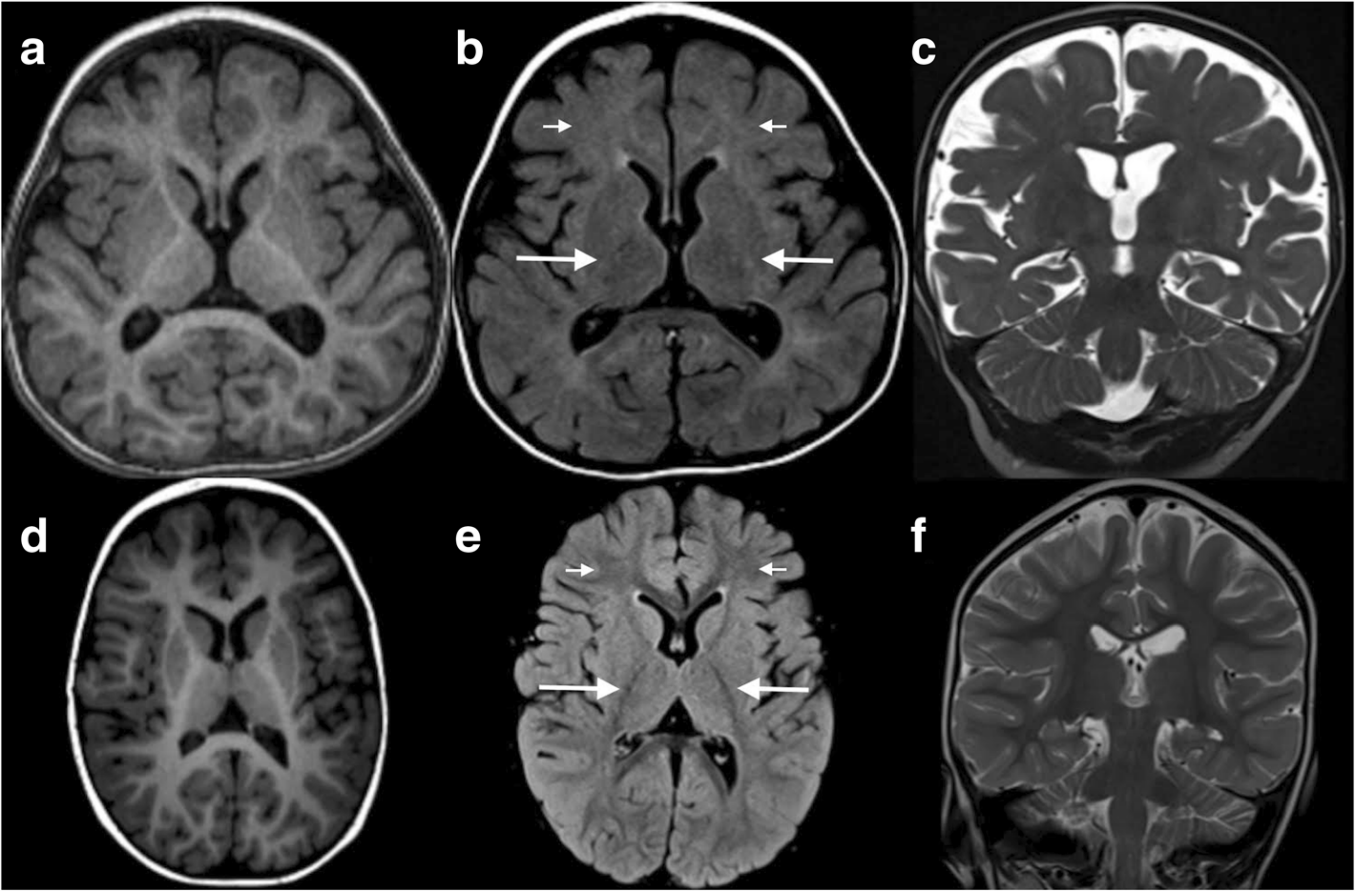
Brain MRI. a-c: SLC35A2-CDG patient at 22 months. d-f: Normal age and sex matched patient for comparison. a, d T1-weighted axial images, b, e TurboFLAIR axial images, c, f T2-weighted coronal images. Images a and b demonstrate mild widening of the cerebral sulci that is most notable in the frontal and temporal lobes. Shortening of the head in the anterior-posterior dimension (brachycephaly) was noted, which may be incidental as there are no other findings to suggest craniosynostosis. Image c also demonstrates cerebral volume loss. Delayed myelination is also best seen on c, exhibiting the lack of T2 hypointensity diffusely in the cerebral white matter (compared to f) expected for age. The delayed myelination is also evident on the TurboFLAIR image b as the lack of characteristic hypointensity in the posterior limbs of the internal capsules (long arrows) and in the deep white matter of the frontal lobes (short arrows)
